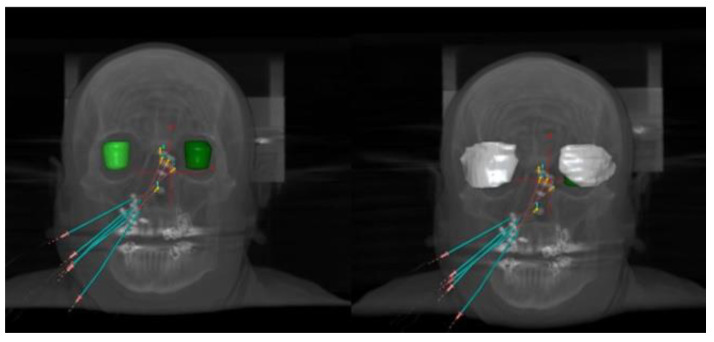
CT of a patient with focus on eyes with and without shielding (with—left/without—right).
